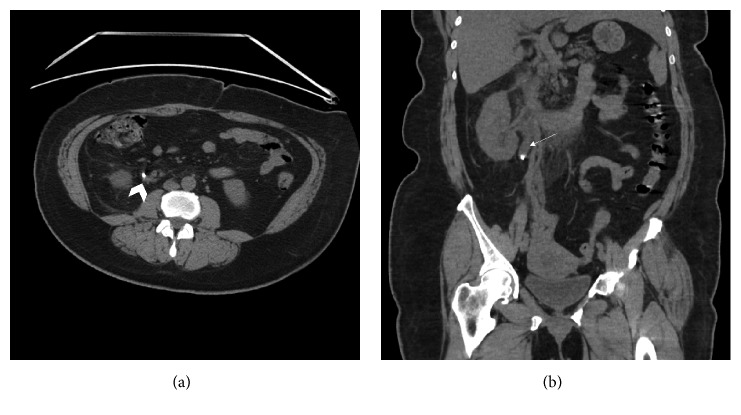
Axial (a) and coronal (b) CT scans of the abdomen and pelvis without contrast revealed an 8 mm right obstructive proximal ureteral stone ((a) arrowhead) causing hydroureter and hydronephrosis ((b) arrow).
